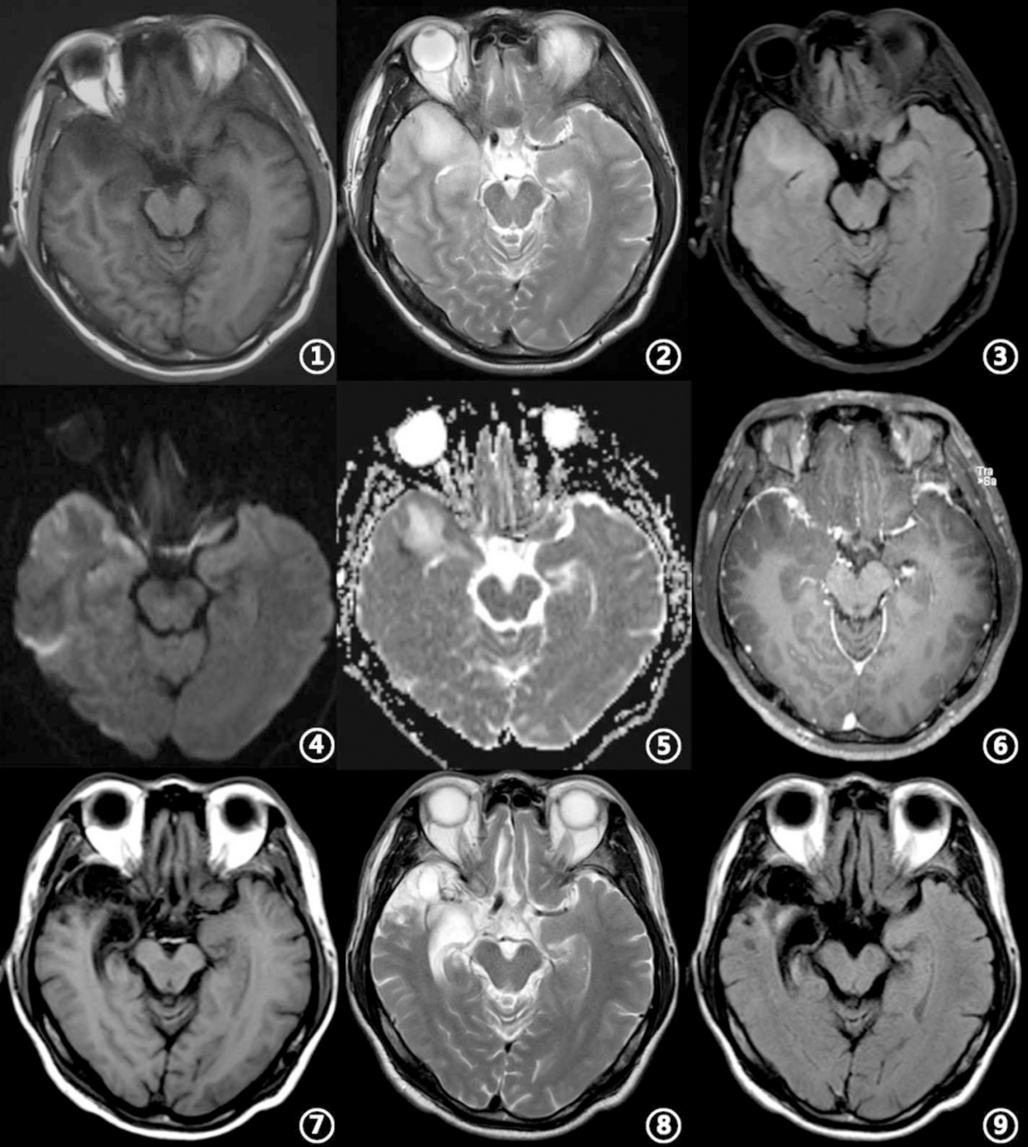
Head MRI results of the patient. ①–⑥ was on admission, and ⑦–⑨ was 1 month later. ① and ② Patchy long T1 signal and slightly longer T2 signal in the right frontal, temporal, and insular lobes; ③–⑤ High signals in Flair and DWI lesion areas, and slightly higher signal in ADC area; ⑥ Patchy enhanced shadows in some lesion areas, and the dura mater adjacent to these lesion areas was enhanced and thickened; ⑦–⑨ Patchy long T1 and T2 signals in the right frontal, temporal pole, and insular lobes, local atrophic, necrotic, and softening foci, and widening of the right temporal horn.
